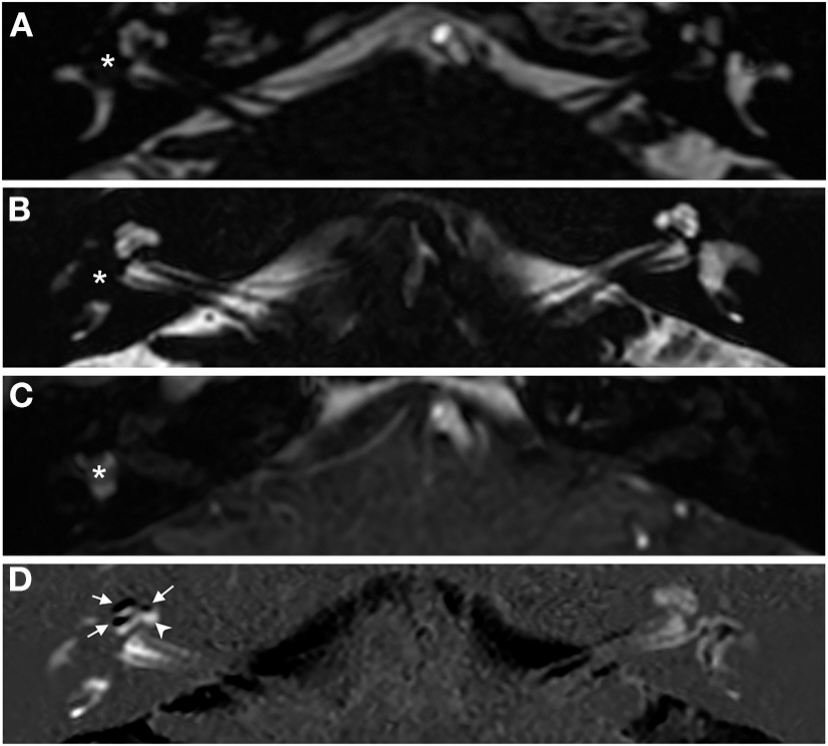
Axial magnetic resonance (MR) images obtained from a patient with concurrent right intravestibular schwannoma and endolymphatic hydrops in the right cochlea. (A) MR cisternography images obtained 4 years after the patient's initial visit to our department. (B–D) MR images obtained 12 years after the patient's initial visit to our department. (A,B) MR cisternography revealed a filling defect in the right vestibule (asterisks). (C) Gadolinium-enhanced T1-weighted imaging showed an enhanced region in the right vestibule indicating the presence of a hypervascular mass (asterisk). (D) Delayed 3D-FLAIR image, subtraction of the positive endolymphatic image from the positive perilymphatic image revealed black areas (arrowheads) representing the right cochlear hydrops and diffuse perilymphatic hyperintense area (arrowhead) indicative of blood–labyrinth barrier impairment.
